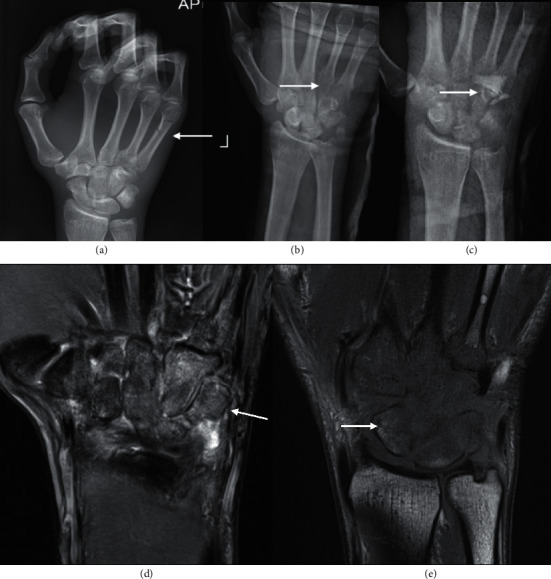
A 45-year-old man involved in a motor vehicle accident sustained an undisplaced 5^th^ metacarpal shaft fracture ((a), arrow). Repeating X-ray 16 days later (b), given the persistent pain, revealed rarefaction of 4^th^ and 5^th^ carpometacarpal (CMC) joints consistent with osteomyelitis and septic arthritis (arrow). X-ray-following washout and introduction of antibiotic-impregnated cement spacer (arrow). Coronal STIR (d) and T1 (e) sequences of the wrist show the extent of the infection involving all carpal bones and base of the 2^nd^ to 5^th^ metacarpals. (c)
